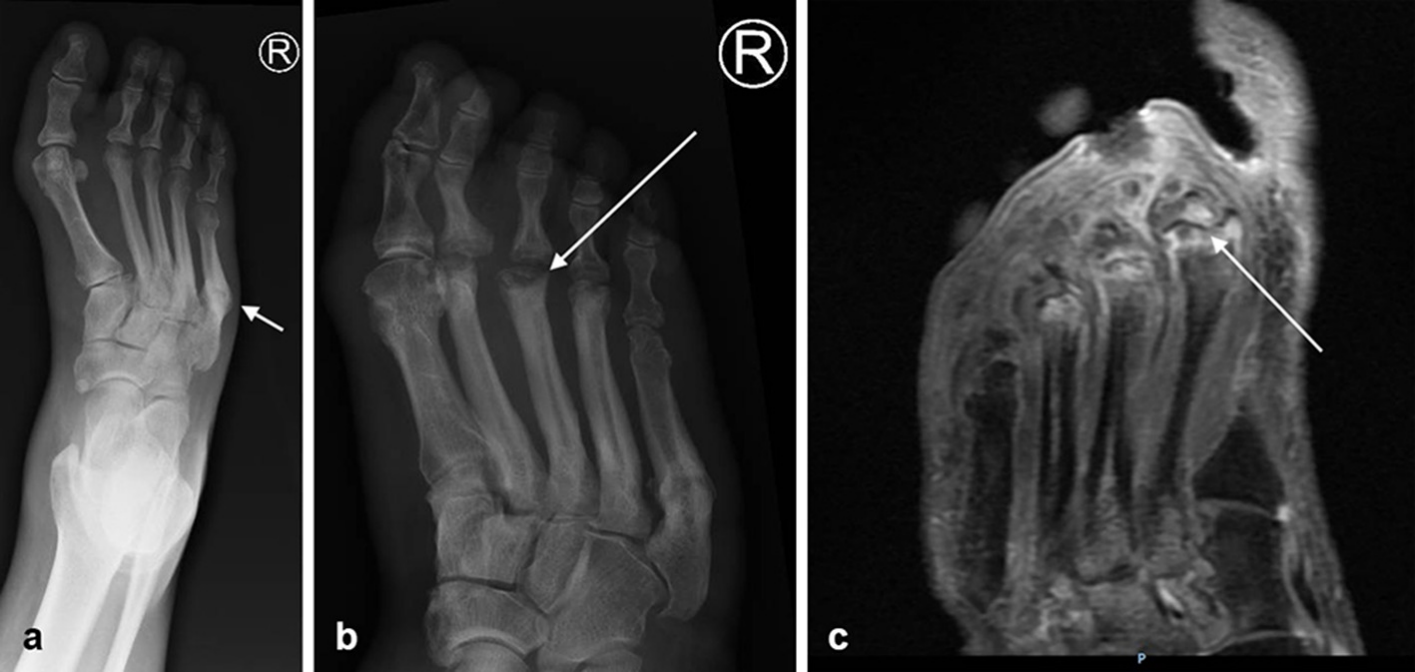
(a) Right foot radiograph 6 months prior to presentation demonstrates an ununited fracture of the proximal shaft of the fifth metatarsal (arrow). Note exuberant callus formation and sclerotic bone margins, likely representing a post fracture pseudarthrosis. (b,c) Right foot radiograph (b) of the same patient performed for investigation of new foot swelling, followed by MRI (c). Radiograph (b) demonstrates collapse and sclerosis of the second, third (arrow) and fourth metatarsal heads. Note associated cortical thickening of the metaphyses and diaphyses. Axial post gadolinium fat-suppressed *T*_1_ sequence (c) demonstrates increased signal in the third, fourth and fifth metatarsal heads and bone marrow oedema with no significant soft tissue involvement, confirming avascular necrosis.
